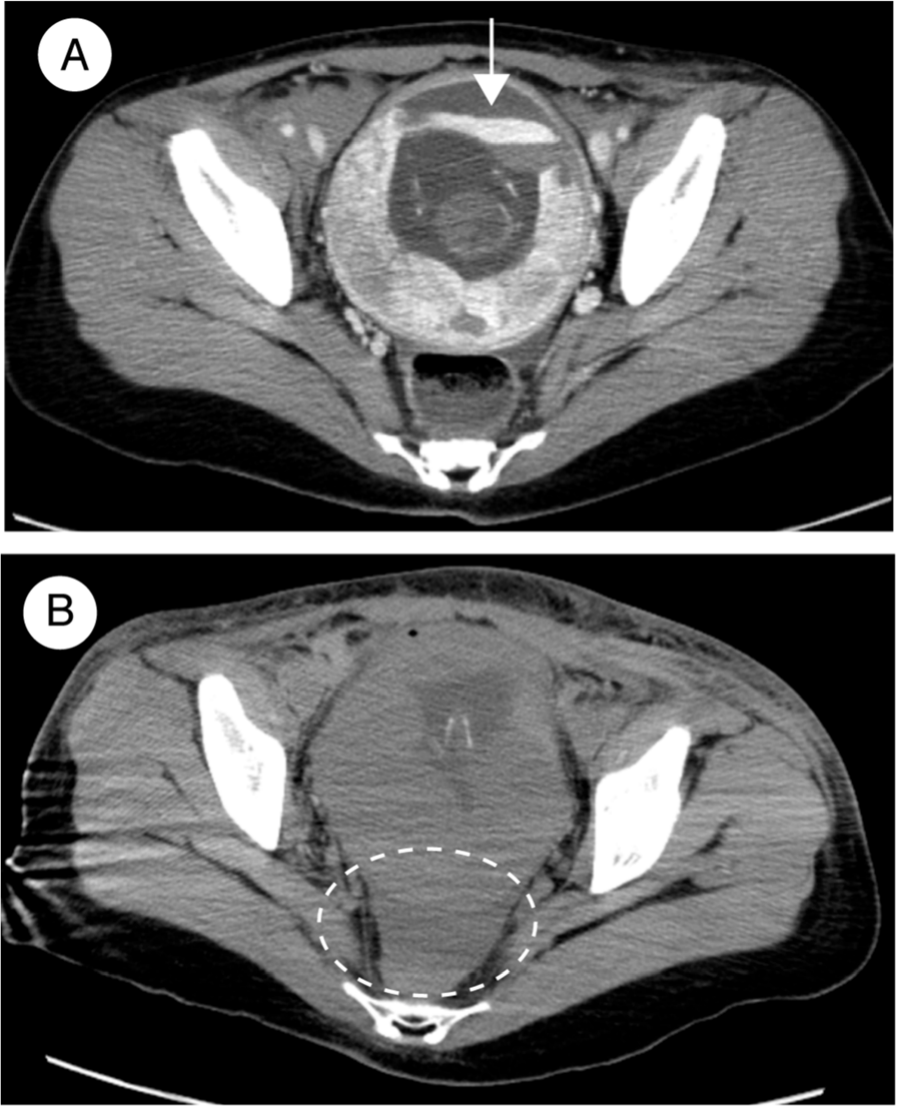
Axial pelvic computed tomography (CT) images at 1 h a and 11 h b after presentation. a Contrast-enhanced CT demonstrating active extravasation into a subchorionic hemorrhage (arrow). b Noncontrast CT demonstrating a retroperitoneal hematoma in the pelvis (dotted circle)
